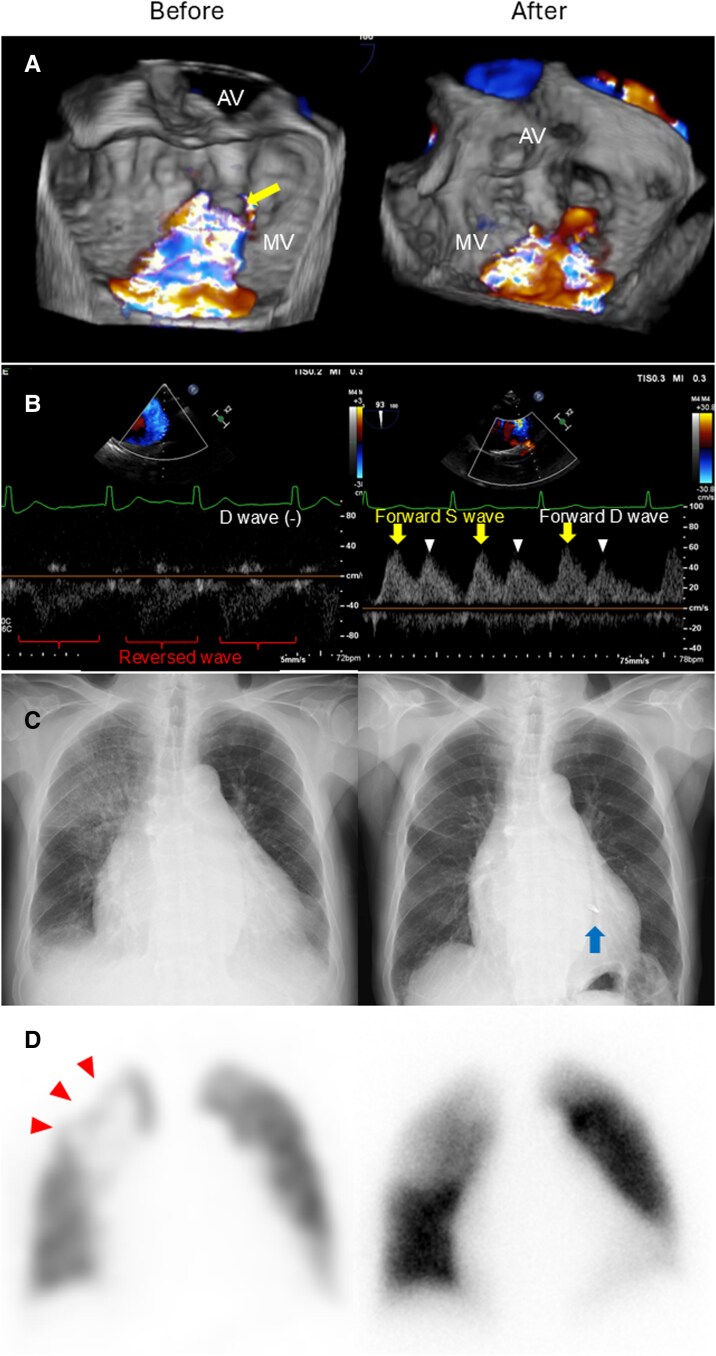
Changes in each imaging modality before and after the transcatheter edge-to-edge repair procedure. (*A*) The mitral regurgitation jet caused by A2 prolapse (left panel, yellow arrow) was divided into two jets by the clip, and its severity was reduced from grade 4+ to grade 2+ (right panel). (*B*) Pulmonary vein Doppler showing restoration of forward systolic (yellow arrows) and diastolic (white arrowheads) waves in the right superior pulmonary vein on the right panel. (*C*) Chest radiograph showing resolution of opacity in the right upper lobe. The clip is shown in the right panel (blue arrow). (*D*) Perfusion scintigraphy showing increased perfusion in the right upper lobe with improvement in the prior defect (red arrowheads). AV, aortic valve; D, diastolic; MV, mitral valve; S, systolic.
